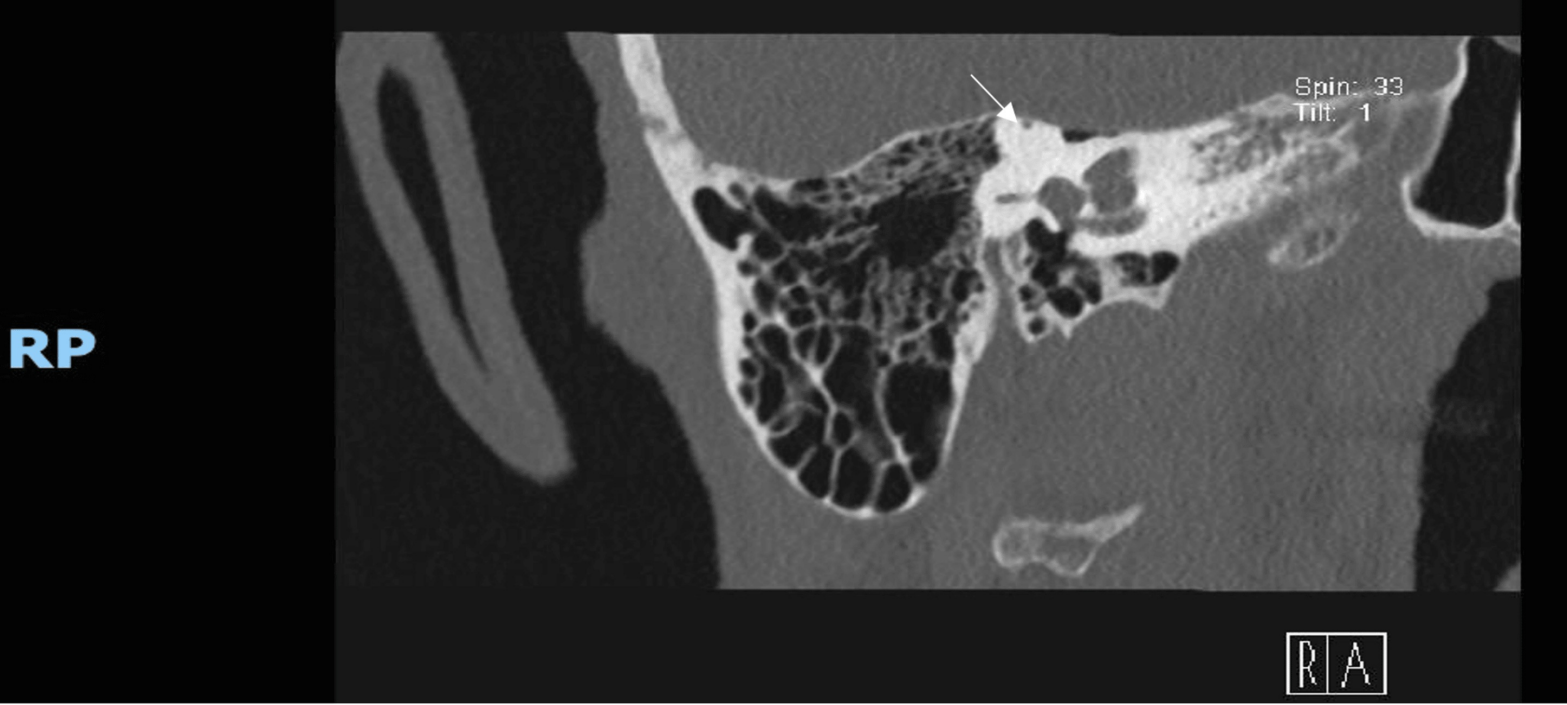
High-resolution coronal CT of the right temporal bone demonstrating clear absence of bony covering over the superior semicircular canal, consistent with right-sided superior canal dehiscence. Note the proximity of the dehiscent canal to the middle cranial fossa, confirming anatomical vulnerability typical of SSCD CT: computed tomography; SSCD: superior semicircular canal dehiscence
